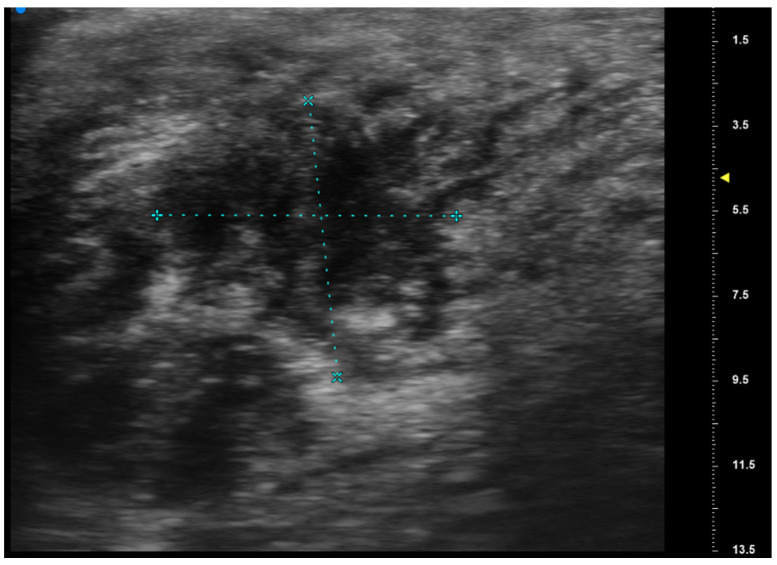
The high-frequency ultrasound depicts an ill-defined hypoechoic lesion located in the oral mucosa. The lesion appears irregular in its contours with deep infiltration into the underlying tissues with dendritic margins. There are coexisting variations in reflectivity and an alteration in the surrounding tissue structure. There are no evident signs of regional metastasis in adjacent structures.
